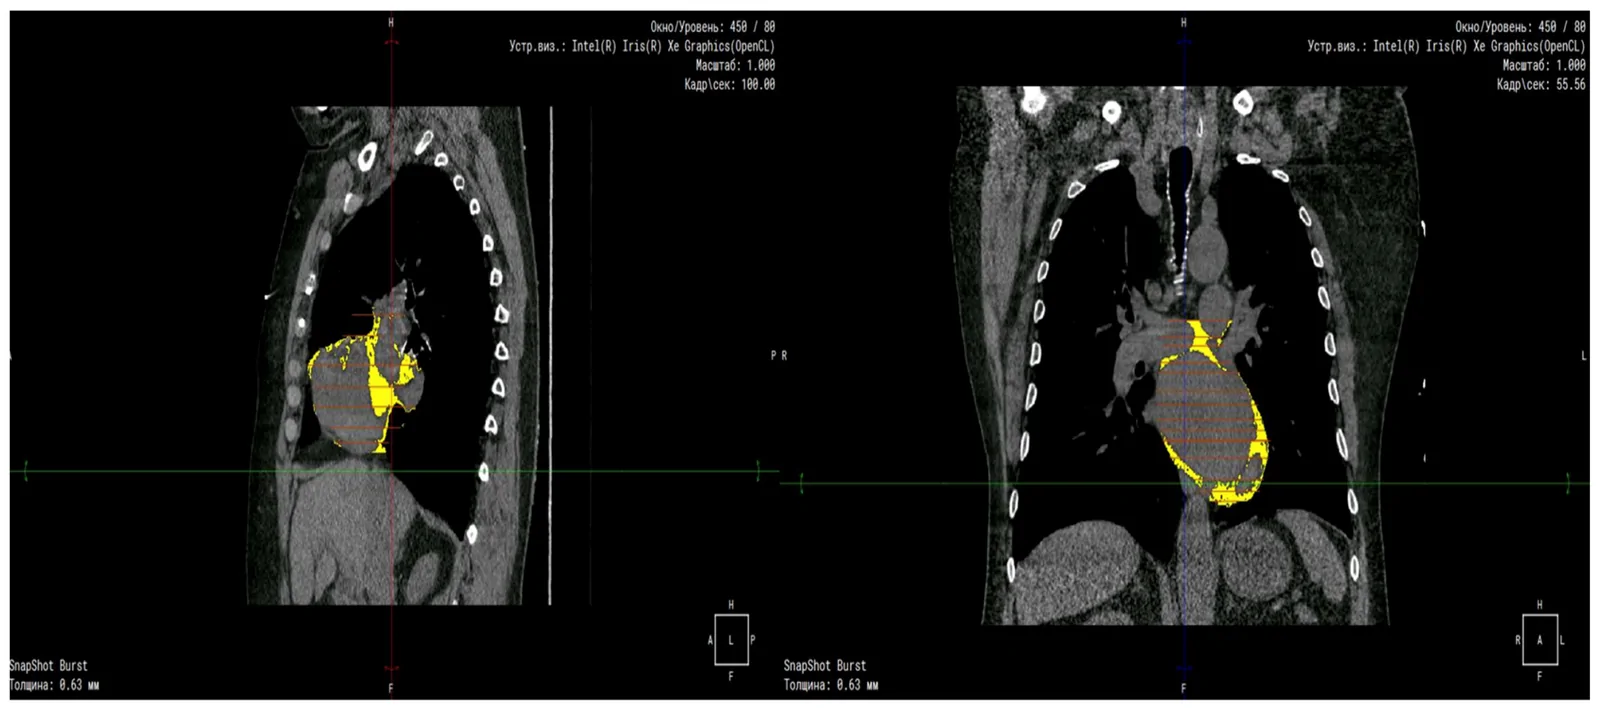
Assessment of epicardial adipose tissue volume using cardiac multislice computed tomography. Note: Epicardial adipose tissue is highlighted in yellow.
